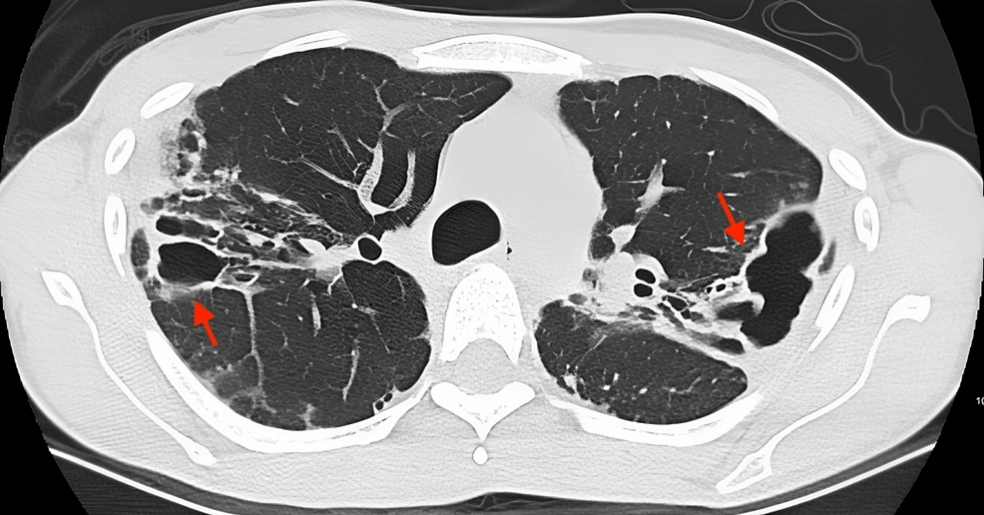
CT chest after the relapse of the infection showing bilateral upper lobes and right middle lobe varicoid and cystic bronchiectasis, demonstrating interval increase in soft tissue thickenings. These findings were consistent with disease progression. CT: computerized tomography
